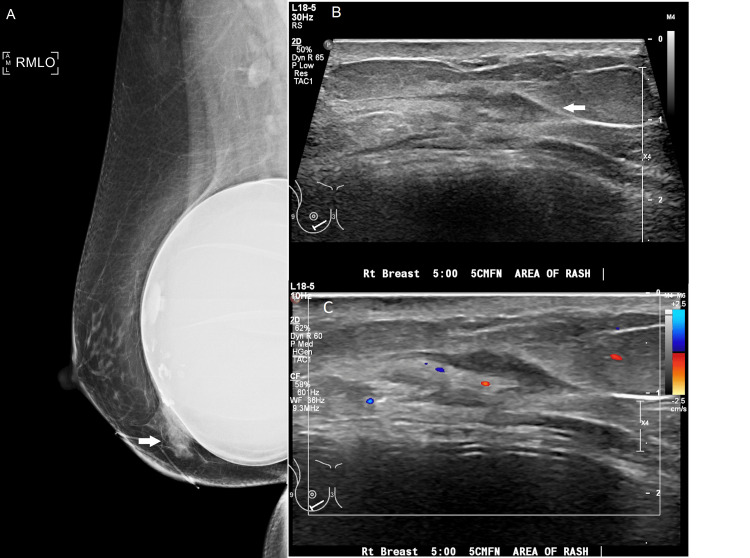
(A) Initial mammogram upon presentation showing an asymmetry (arrow) inferiorly with adjacent focal skin thickening. (B) Grayscale ultrasound image demonstrating nonspecific hypoechoic tissue (arrow) at the site of asymmetry on mammogram. (C) Color Doppler ultrasound image showing normal vascularity throughout the area of concern.
